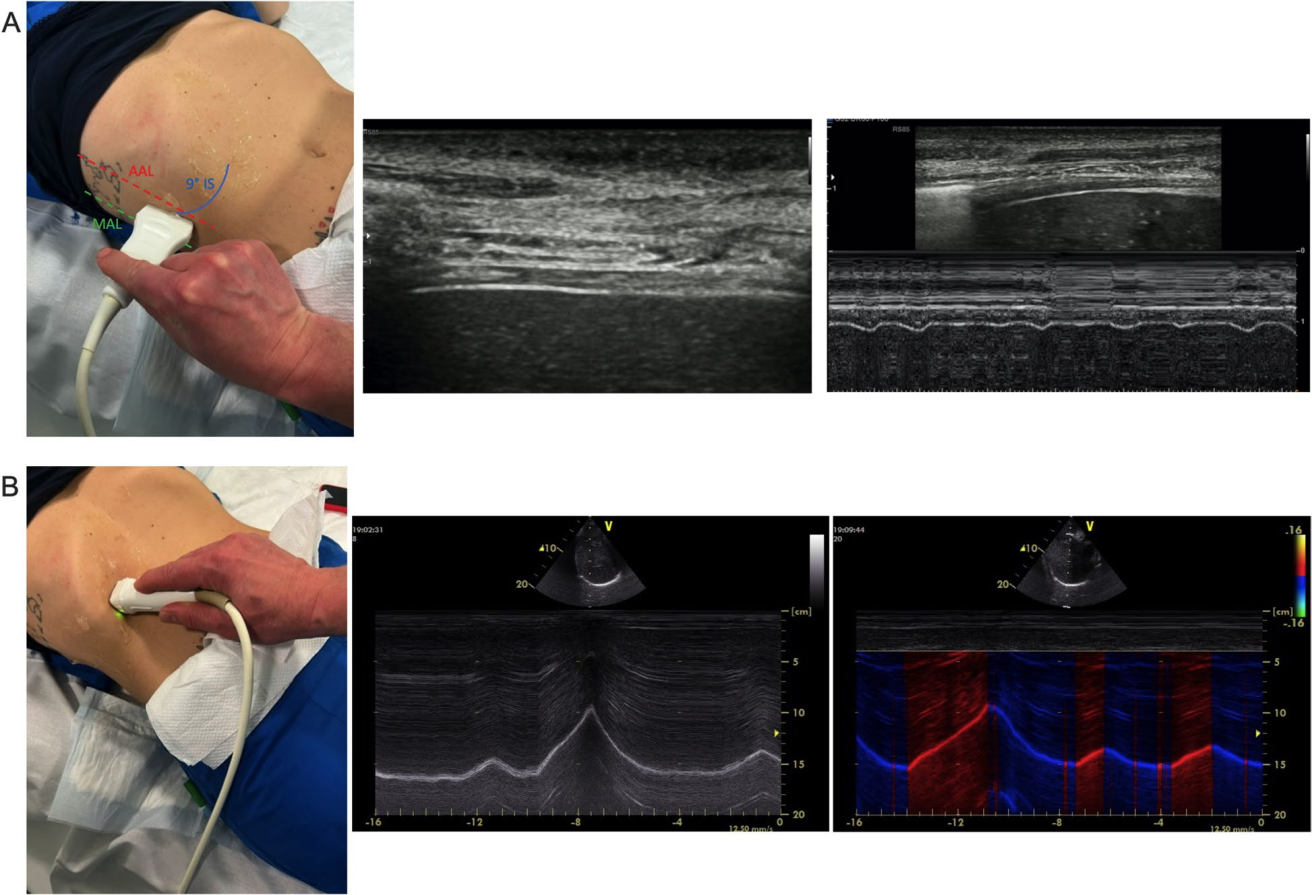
Diaphragmatic ultrasound. Diaphragmatic ultrasound for thickness (A) and excursion (B) are depicted. A Diaphragmatic is described as a three-layers structure involving a hypoechoic stratum between two hyperechoic edges, i.e., external pleural line and internal peritoneal lines; diaphragmatic thickness changes at varying phases of respiratory cycle. B Diaphragmatic displacement variations in response to respiratory cycle. Red, inspiratory excursion; Blue, expiratory excursion
